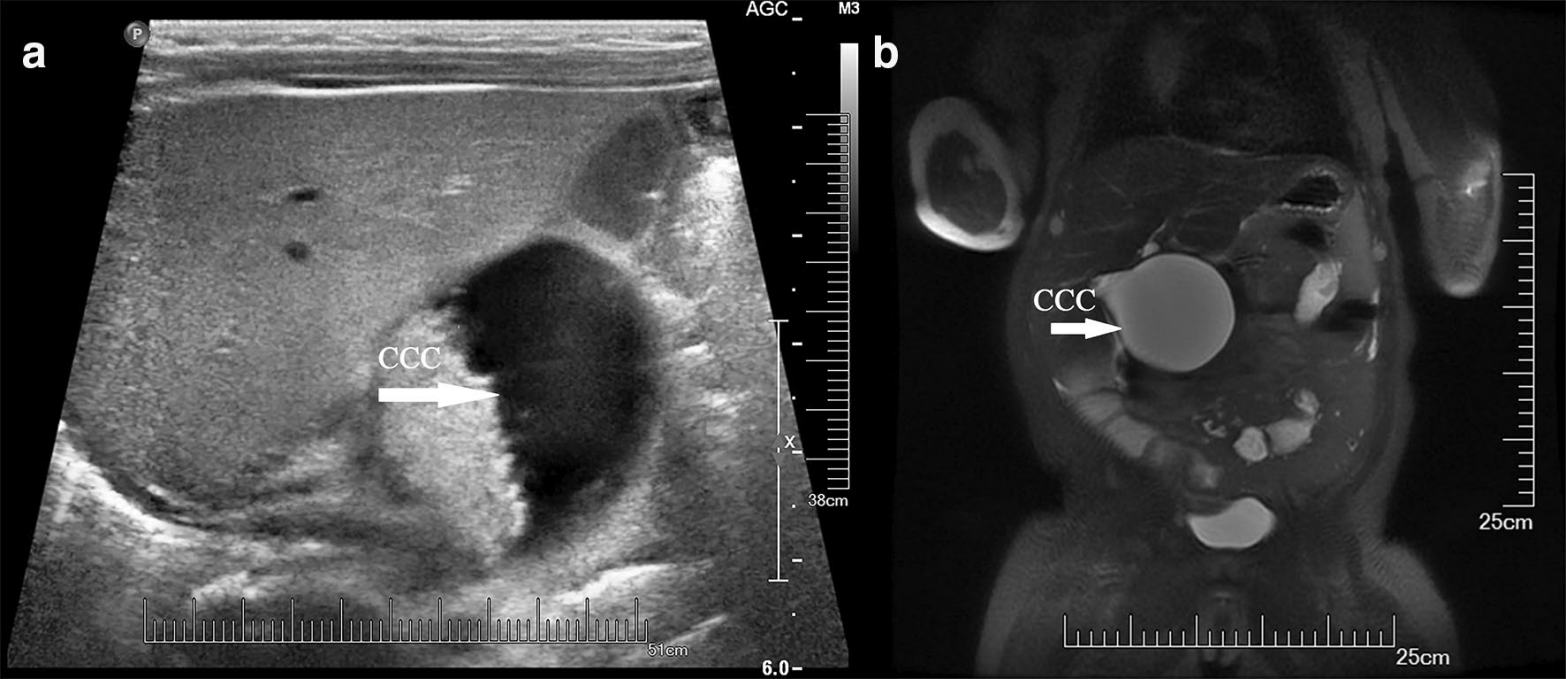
The common bile duct showed cystic dilatation (CCC) on US (a) and MRI (b) in a prepartum diagnosed CM patient after birth.
